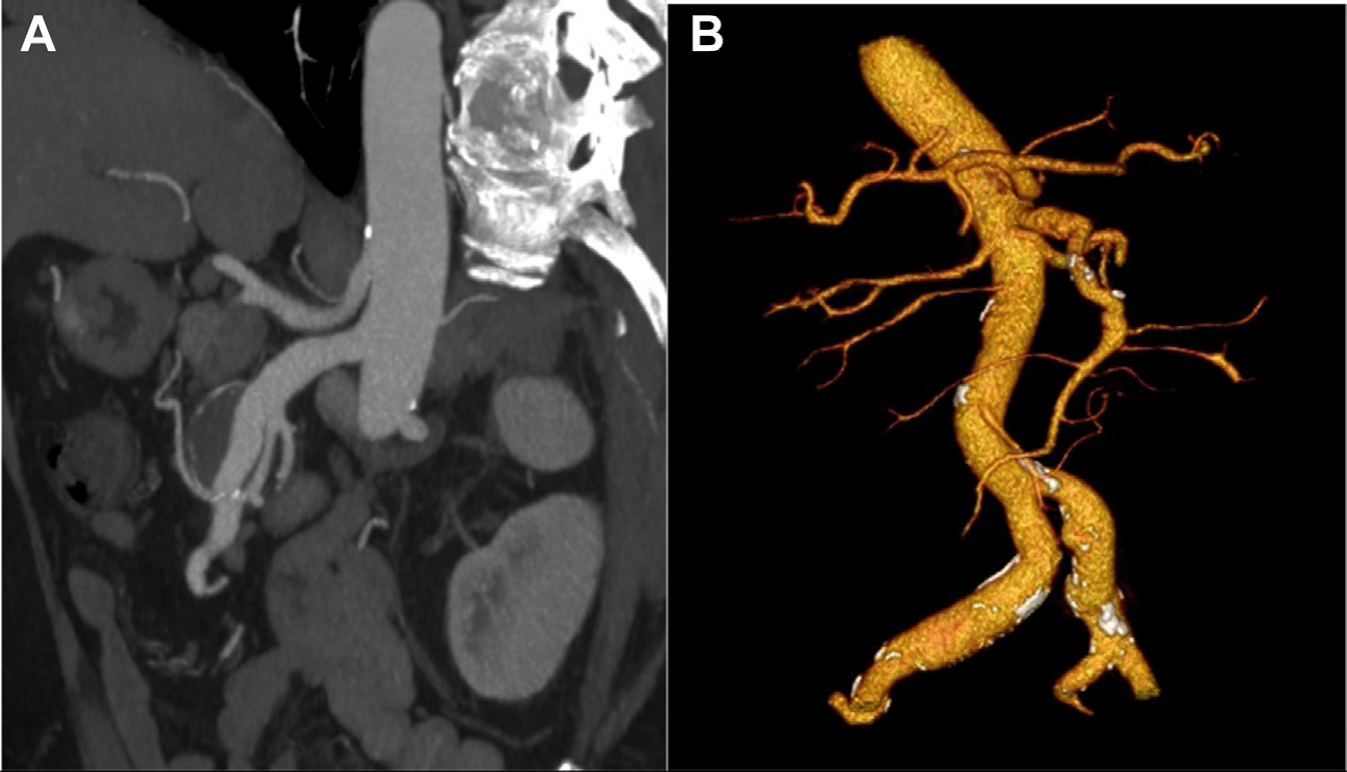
Case 2: Preoperative and Postoperative Imaging of Superior Mesenteric Artery Reconstruction Using Superficial Femoral Artery Graft (A) Preoperative computed tomography angiography showing an aneurysm of the superior mesenteric artery. (B) Postoperative computed tomography volume rendering of the superior mesenteric artery reconstruction with a superficial femoral artery graft.
